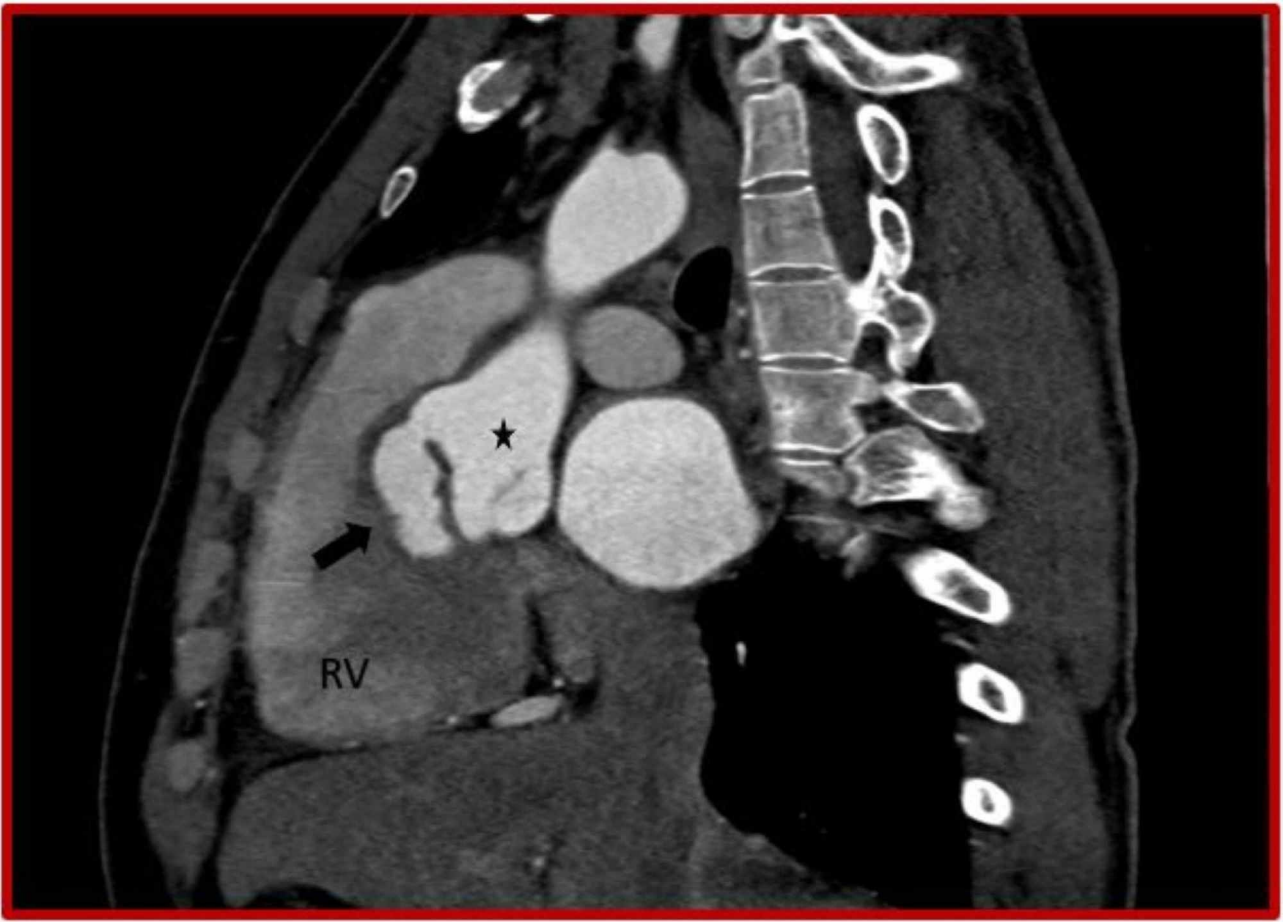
Cardiac CT (sagittal oblique view) demonstrates ruptured right sinus of Valsalva aneurysm (asterisk) dissecting into the interventricular septum (black arrow). CT: Computed tomography; RV: Right ventricle.
